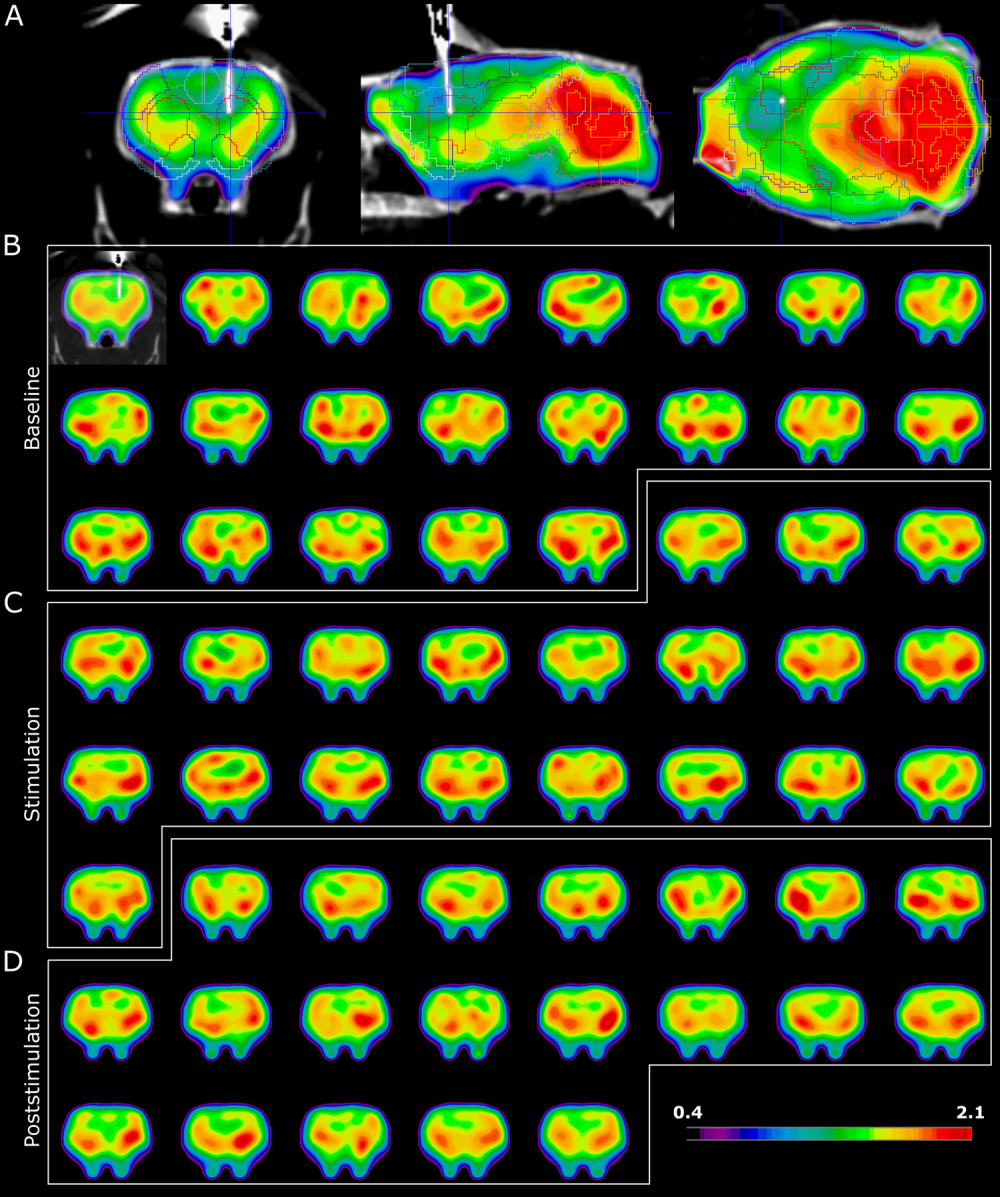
(A) Position of the fiber tip in the right DS during the scan, here shown as the first 20 minutes average PET + anatomical CT image with overlayed volumes of interest. (B-D) First image shows the position of the implant on the 20 minutes average image, followed by 1 minute dynamic time frames from the same coronal slide, showing the temporal evolution of the tracer activity before (B), during (C), and after (D) the stimulation.
